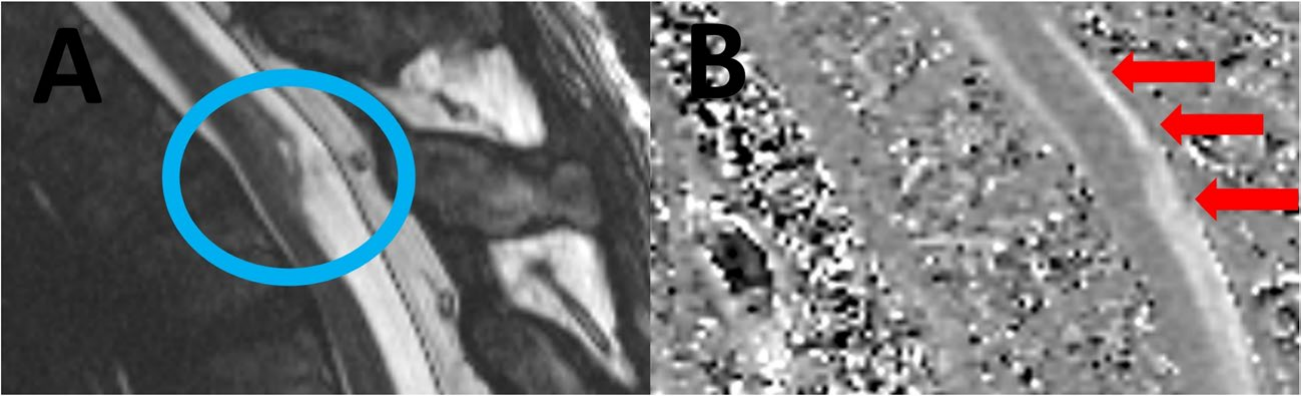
Preoperative sagittal T2-weighted magnetic resonance images (A) and CINE (B) revealed displacement and caliber variation of spinal cord (scalpel sign) (blue circle), and remnant CSF flow (red block arrows), without any signs of a archnoid cyst.
